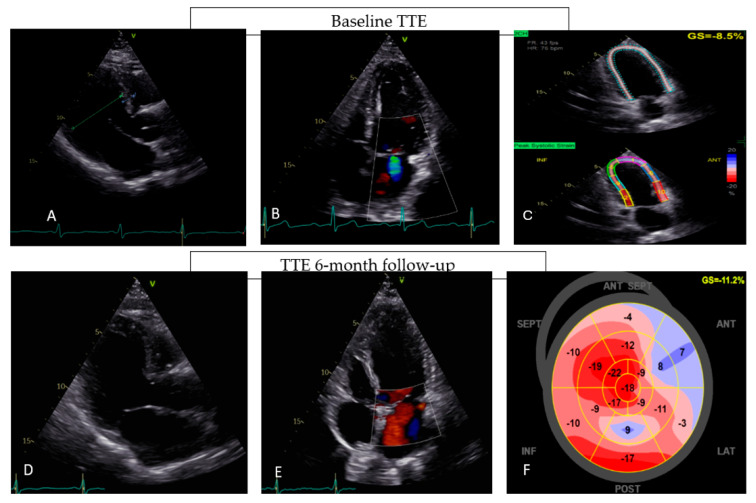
Baseline and 6-month follow-up TTE. Legend: (A). Parasternal view, enlarged left ventricle (62 mm); (B) apical 4-chamber view, mild mitral regurgitation; (C) global longitudinal strain 2 chamber; (D) parasternal view (at 6 months), modest improvement in ventricular geometry; (E) apical 4-chamber view showing no mitral regurgitation; (F) bull’s eye global longitudinal strain evaluation.
